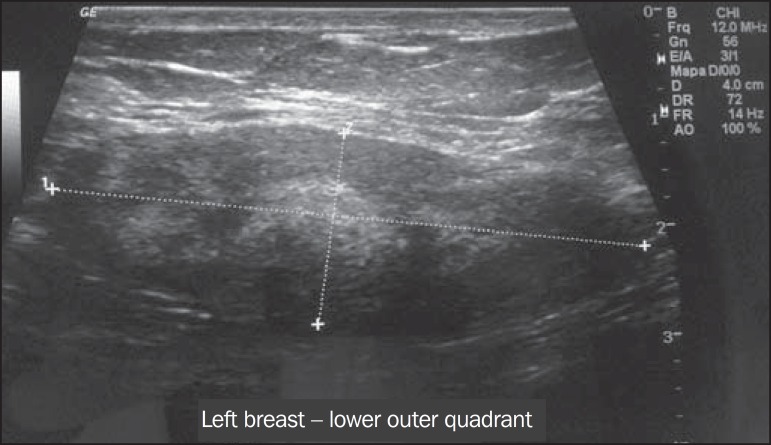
Ultrasound examination of the left breast showing a hypoechoic oval nodule
